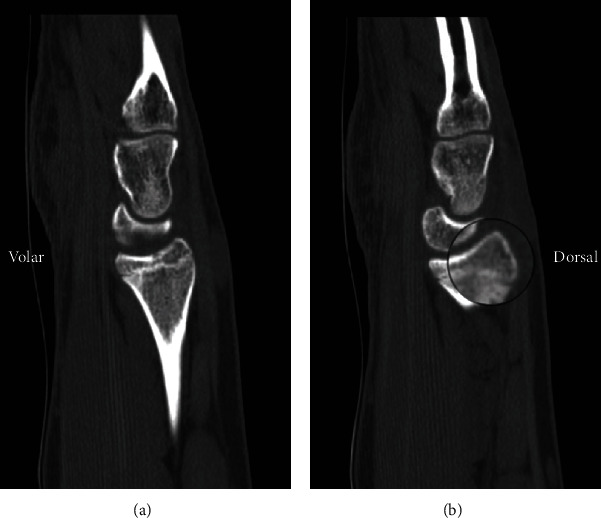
Sagittal CT view showing intra-articular extent of the fracture through the dorsal rim of the radius with interval healing.
